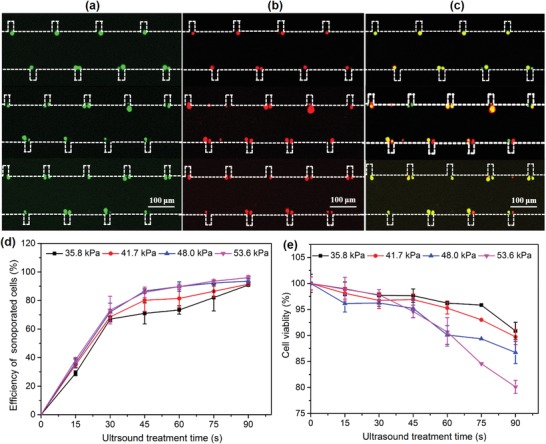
a) Single cells trapped at the oscillating microbubble array emit green fluoresce, confirming the cell viability. b) Trapped single cells emit red fluoresce under the action of oscillating microbubble array. c) Merged fluorescence image showing that high sonoporation efficiency can be achieved by a parallel stable cavitation microbubble array. d,e) Sonoporation efficiency and cell viability at various acoustic pressures as a function of ultrasound treatment time.
